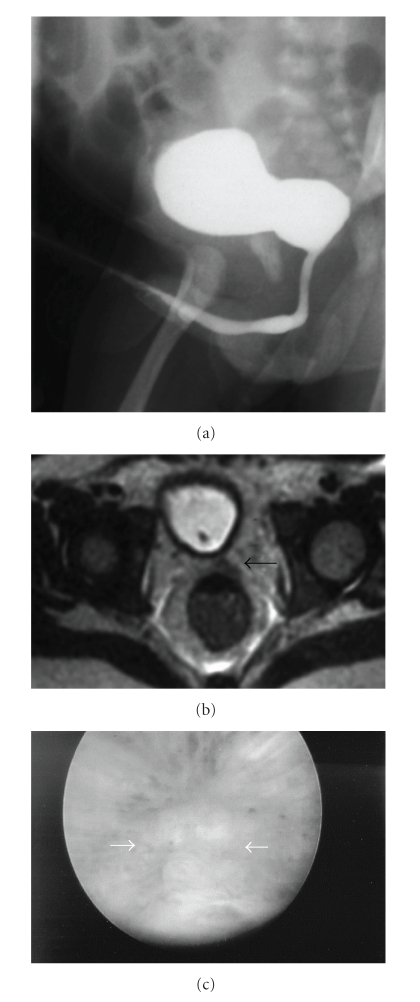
Voiding cystourethrography, magnetic resonance imaging, and urethroscopy in prune-belly syndrome. (a) In a 2-month-old child, the voiding phase of cystourethrography shows a large bladder neck without VM salience in the posterior urethra. (b) In the same patient at the age of 10, T2-weighted axial scan shows the absence of the right deferent and seminal vesicle, while the left seminal vesicle is tubular (black arrow) and the prostate gland is not detected. (c) Urethroscopy confirms the verumontanum hypoplasia, which is quite appreciable (arrows).
